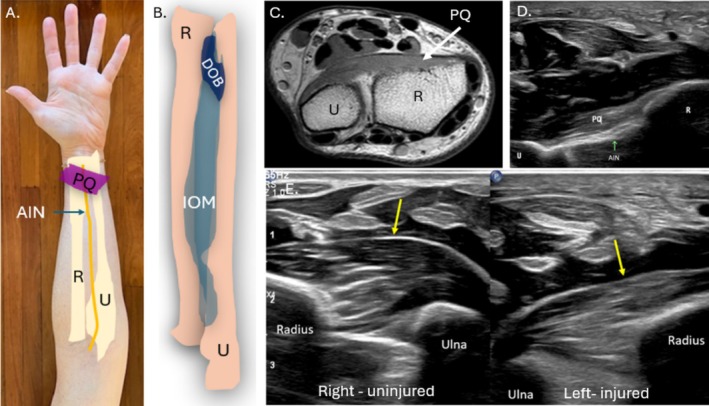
(A) Position of the pronator quadratus (PQ) muscle and the anterior interosseous nerve (AIN) in the anterior forearm. (B) The interosseous membrane (IOM) runs between the radius (R) and ulna (U). The distal end of the IOM is the thickened distal oblique bundle (DOB) which attaches to the dorsal rim of the sigmoid notch of the radius. (C) Axial magnetic resonance (MR) image of the distal forearm demonstrating the PQ muscle. (D) Short axis sonographic image of the distal anterior forearm, demonstrating the PQ muscles (yellow arrow) and the AIN deep to it. (E) Comparison short axis images of the distal anterior forearm, demonstrating the PQ muscle in uninjured and injured limbs. The left forearm and radius had been fractured and the AIN was entrapped by fracture fragments causing denervation and subsequent atrophy and increased echogenicity of the PQ muscle.
